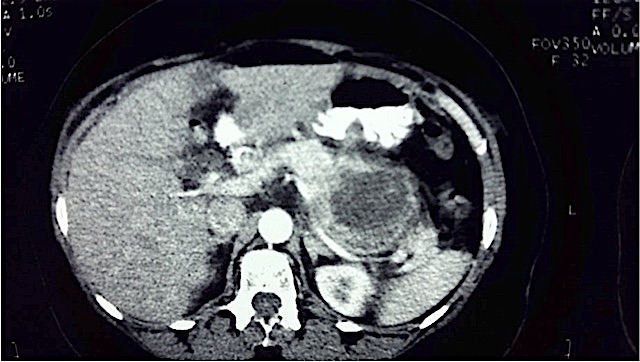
Axial abdominal contrast-enhanced computed tomographic scan showing a large tumour in the region of the body of the pancreas
